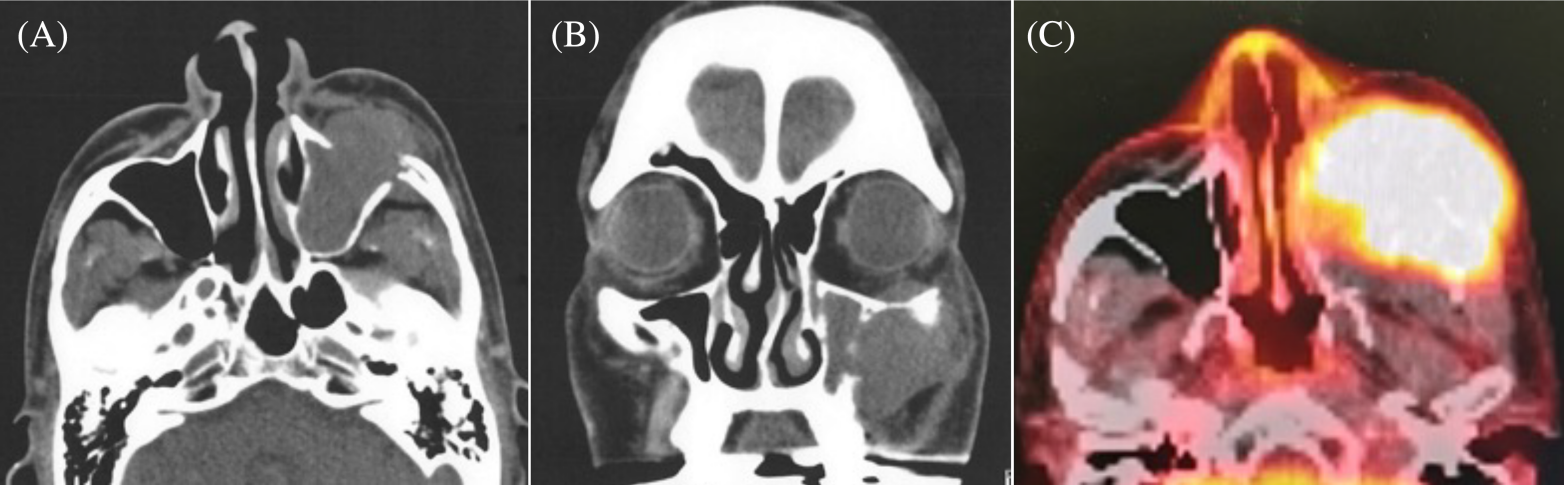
Computed tomography (CT) at initial presentation. (A) Axial view showing a tumor occupying the maxillary sinus with bony destruction of the anterior wall. (B) Coronal image showing intraocular extension with bone destruction in the superior and lateral walls. (C) Positron emission tomography/CT showing intense uptake in the same lesion.
